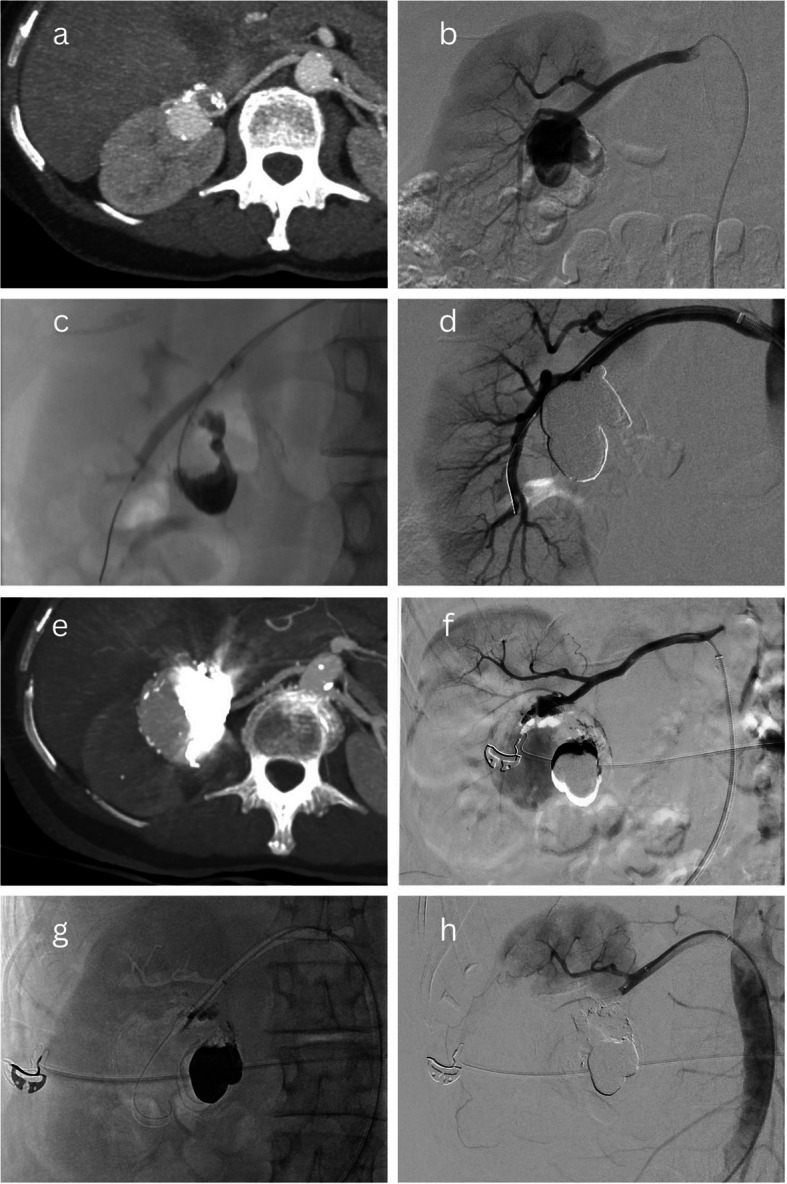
Balloon-assisted Onyx embolisation– Reintervention case 1. A 35-mm saccular Type 2 Rundback aneurysm with two efferent branches (a-b) was treated endovascularly by catheterising the aneurysm sac and separately manipulating a wire across the aneurysm into an efferent branch and positioning a balloon across the aneurysm’s origin (c). The balloon was inflated and the sac was filled with Onyx until successful exclusion (d). Four years later, the patient presented acutely with right loin pain and haematuria; CT and angiography demonstrated reperfusion and enlargement of the aneurysm to 56 mm (e-f). Successful reintervention was performed in an emergent setting by manipulating a wire into the aneurysm sac and embolising the sac and the aneurym’s neck with multiple endovascular occlusion systems and vascular plugs (g) at the expense of approximately 66% of renal parenchymal perfusion (h). Over time, this kidney became atrophic and non-functioning but biochemical renal function remained within the normal range
